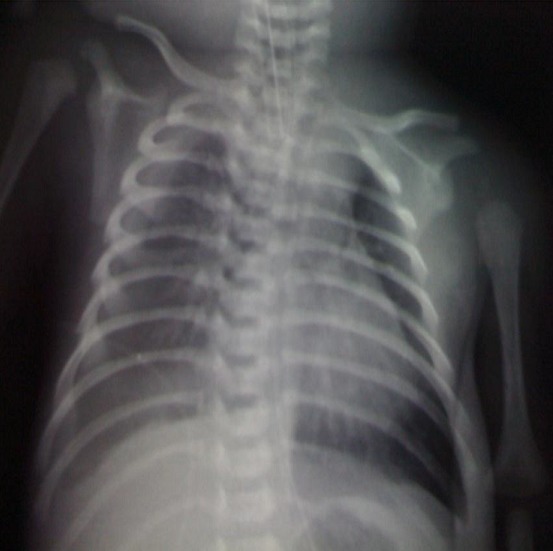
Radiographie du thorax montrant un épanchement pleural droit de grande abundance
